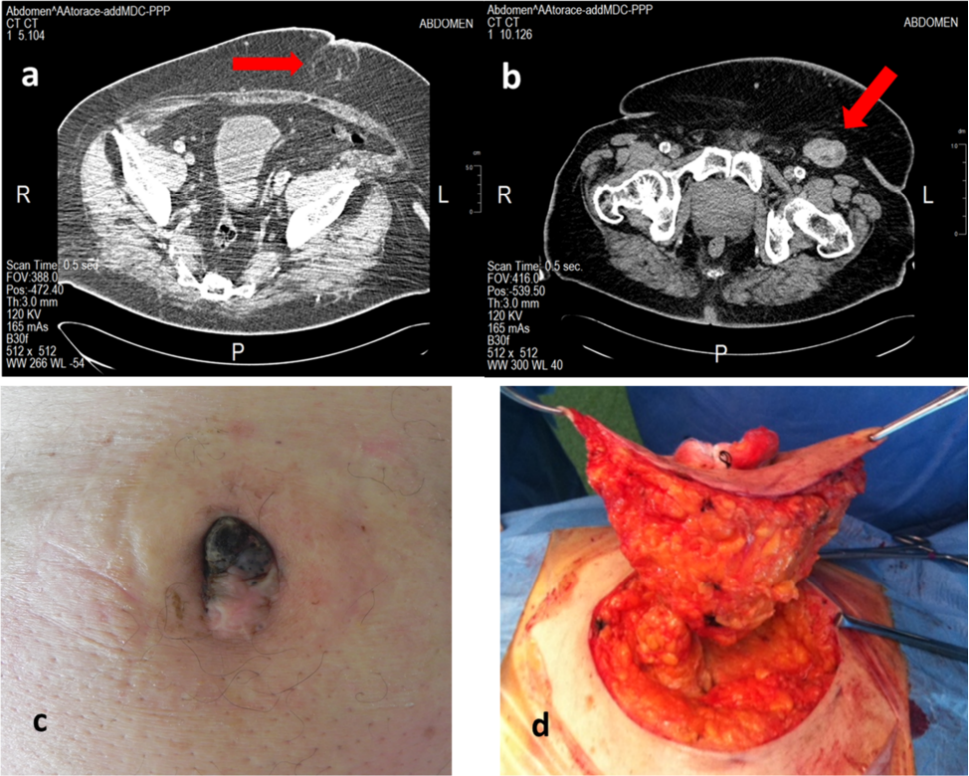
Case 3. (a) Computed tomography image showing nodular swelling in the umbilical region (b) Computed tomography image of left groin lymphadenopathy of 54 x 28 mm (c) pre-operative view (d) wide excision comprising the underlying peritoneum.
